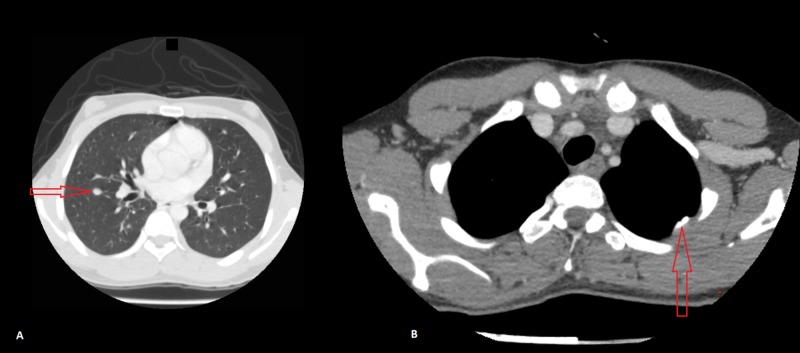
Contrast enhanced high resolution computed tomography (HRCT) scan of the chest. Image A: Red arrow points toward a calcified nodule in the right lung (likely metastatic). Image B: Red arrow points toward a pleural-based nodule (likely metastatic).
